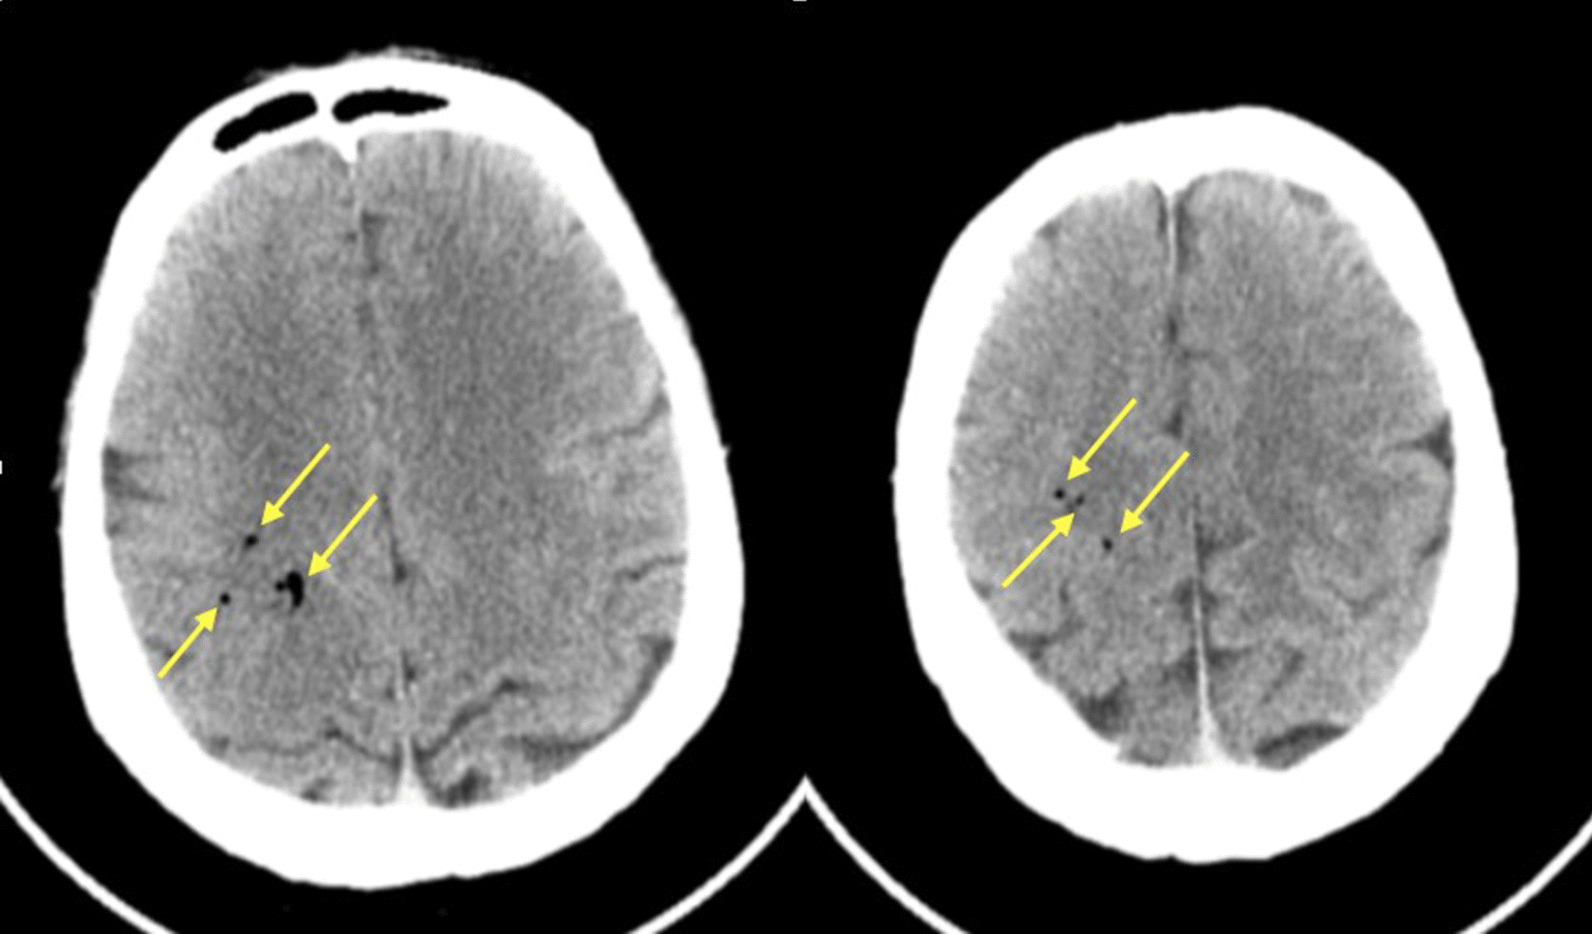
The cerebral CT scan following bronchoscopy showed multifocal cerebral air embolisms in the right frontal lobe (arrows)
